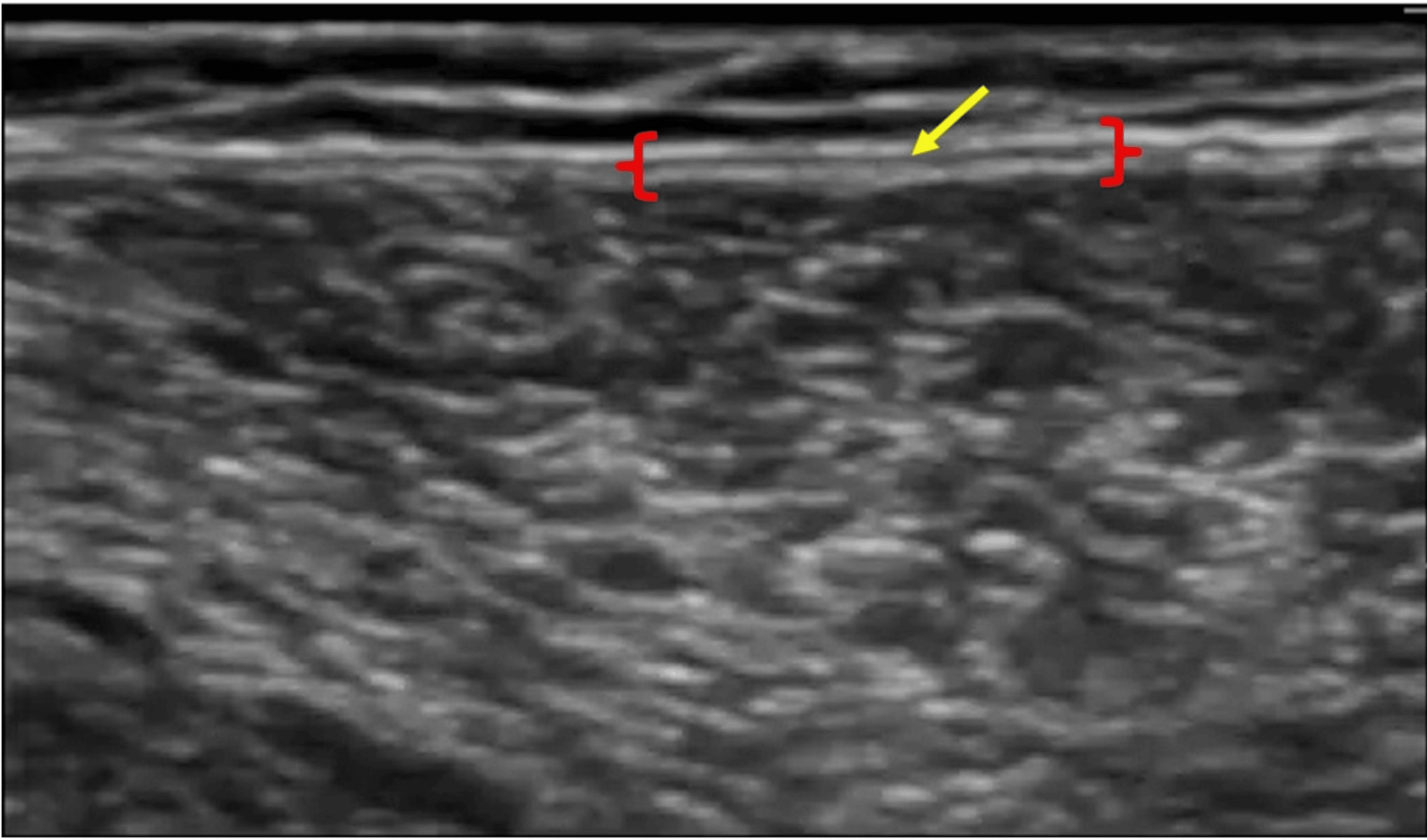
Two close fascial layers in the distal part of the fascia lata Two close fascial layers in the distal part of the fascia lata Arrow: the tip of the needle for injection. {}: two close fascial layers. Note: this is a short-axis ultrasound image.
